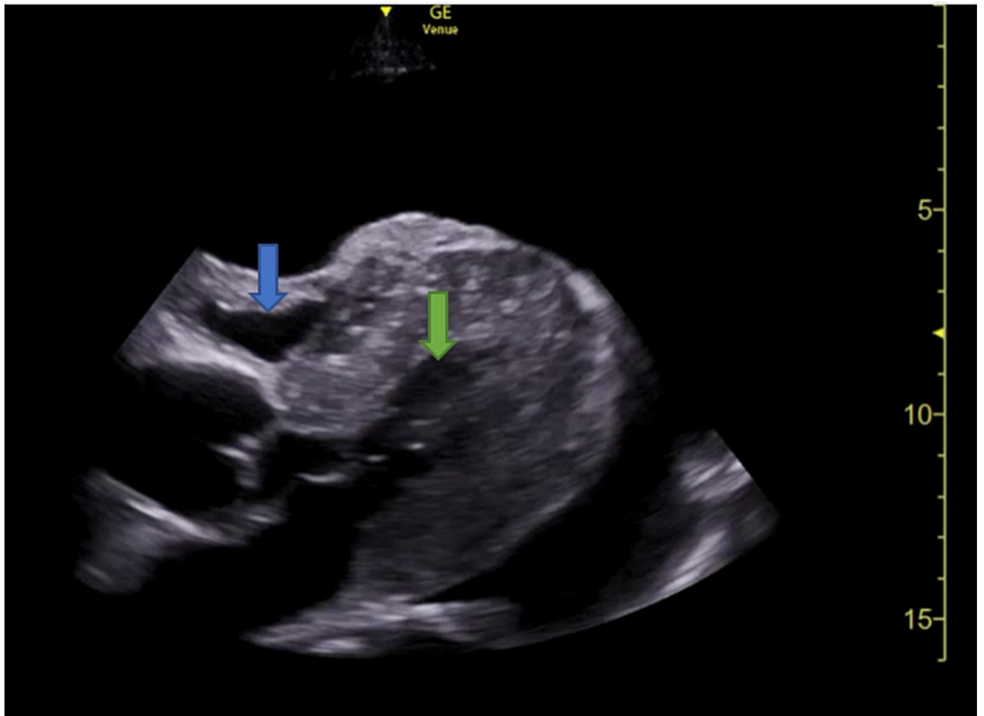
Echocardiography showing right ventricular collapse during diastole Blue arrow: right ventricle. Green arrow: left ventricle
